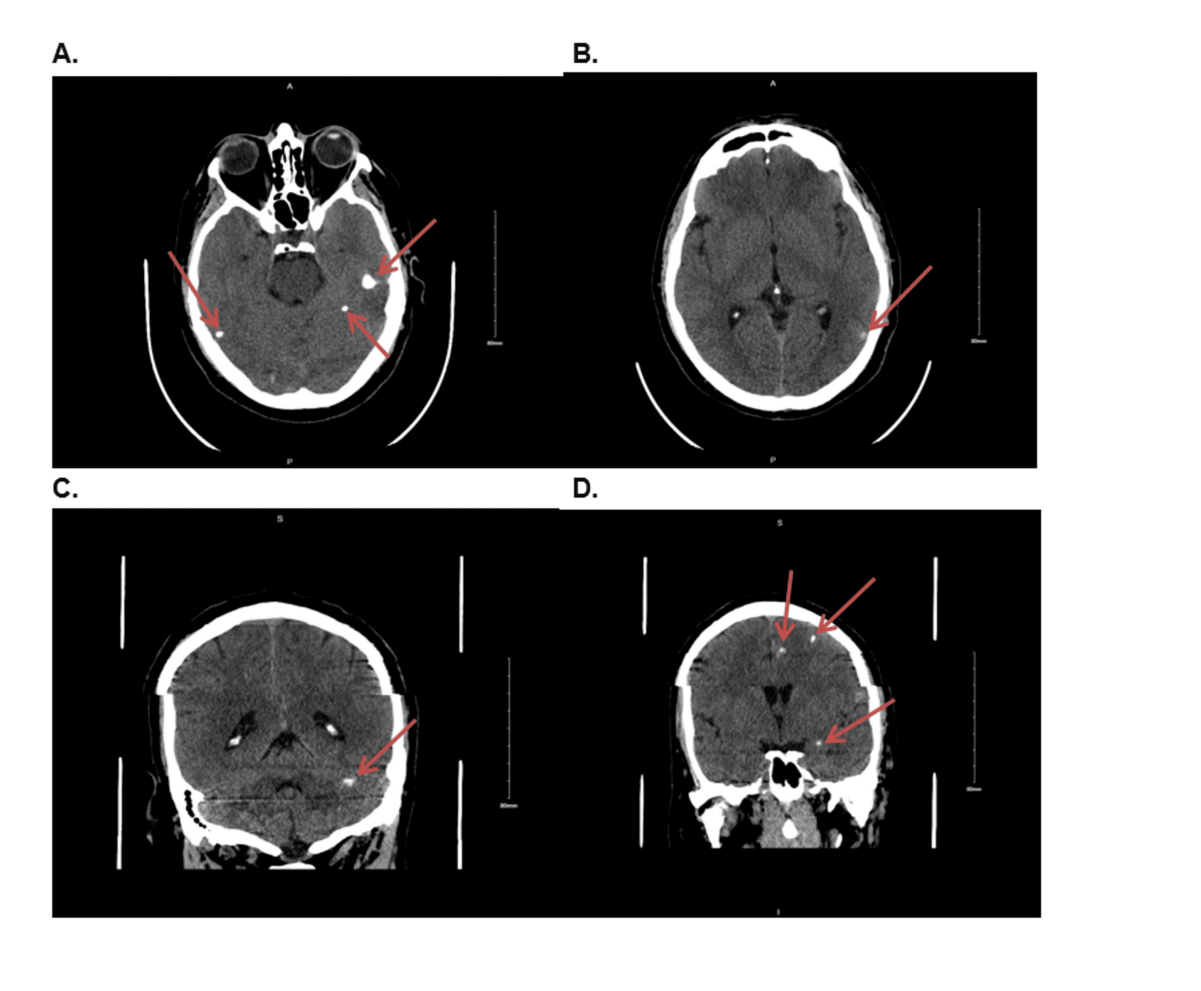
A-D: CT head without contrast showing multiple partially calcified lesions throughout the brain. The lesions appear hyperdense, representing calcified, nonviable cysts, which correspond to the late stage of neurocysticercosis.
